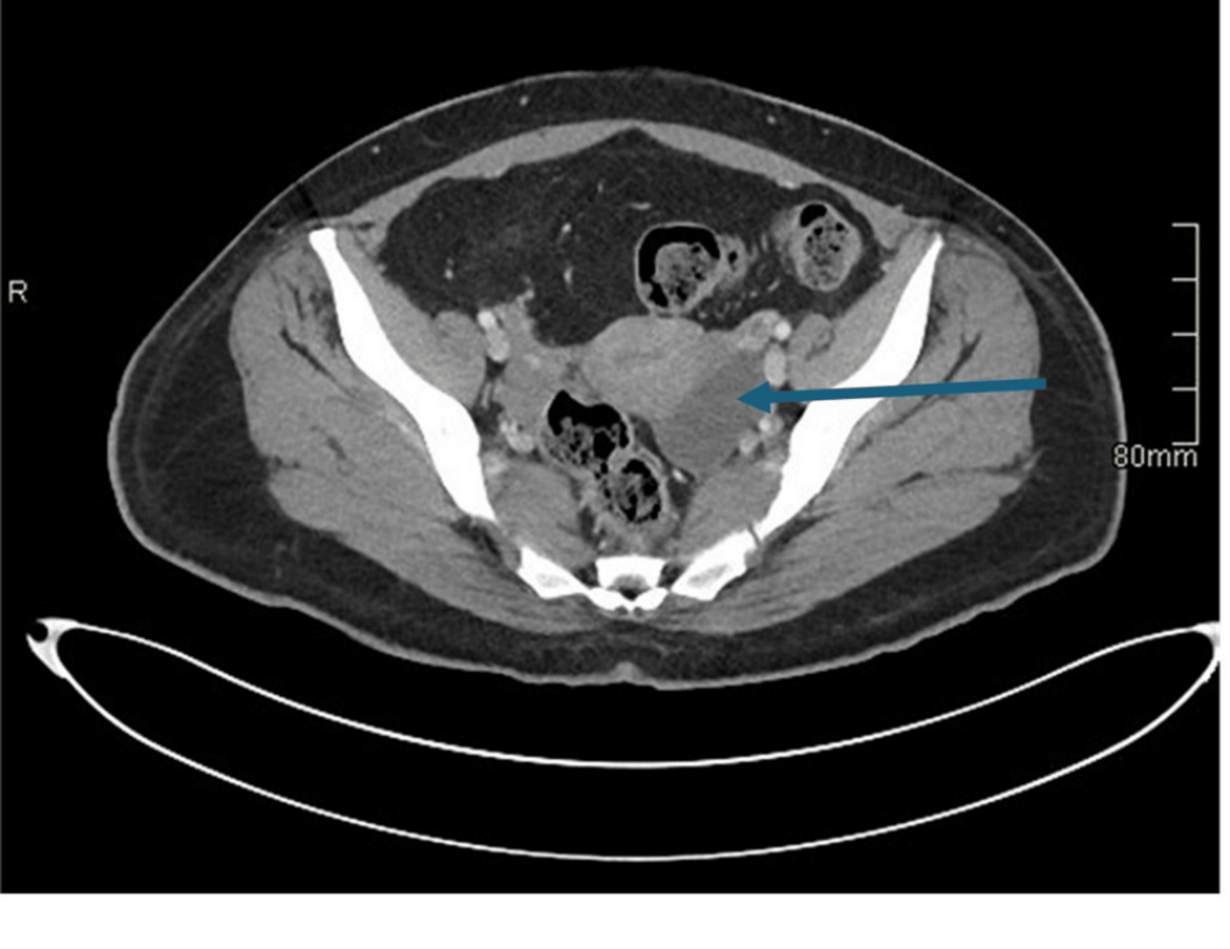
CT of the abdomen and pelvis with contrast redemonstrating her left ovarian cyst (blue arrow) in an axial plane
